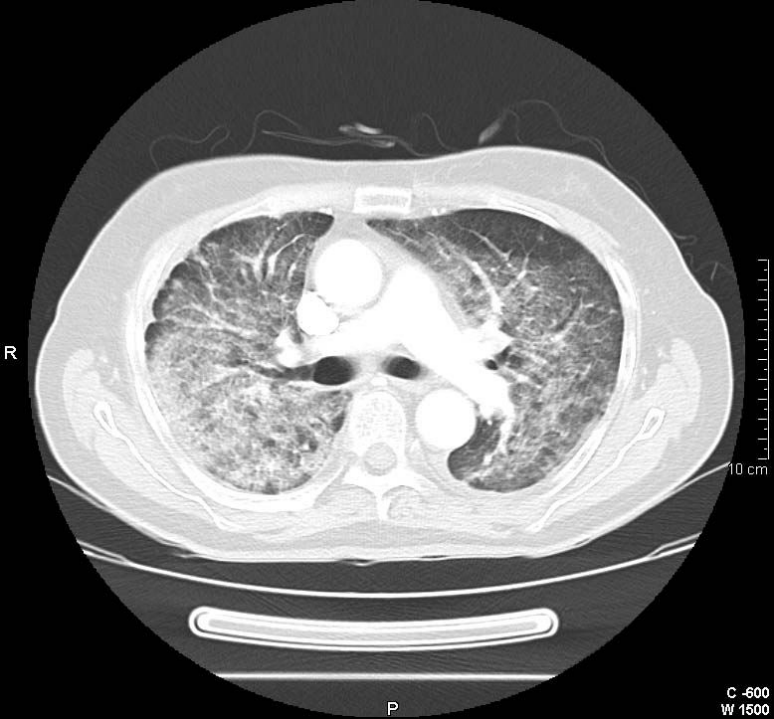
Pulmonary CT scan shows extensive ground glass opacities.
